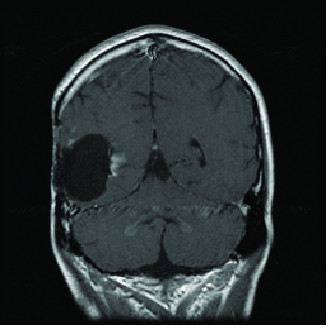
MRI six months following fourth course of radiation therapy. Improvement is shown in intensity of enhancement of the temporal lesion.
